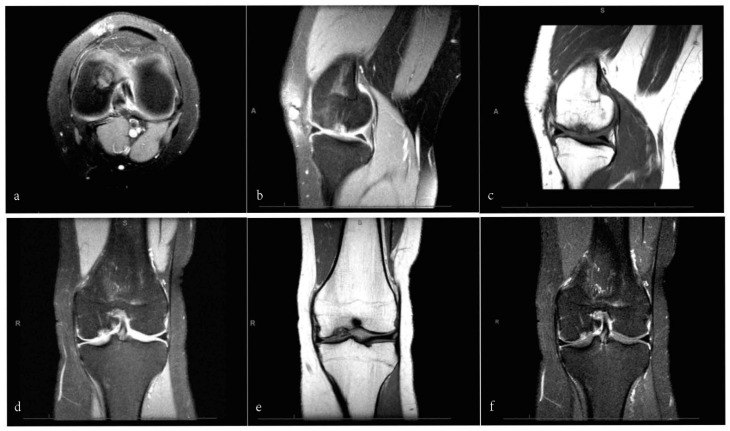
MRI sequences of a focal chondral defect of the medial femoral condyle in a 28-year-old male patient: axial (a) and sagittal (b) views using a proton density turbo spin-echo SPIR sequence; sagittal (c) and coronal (e) views of T1-weighted SPIR sequence; coronal view of proton density turbo spin-echo (f) and with SPIR sequence (d).
